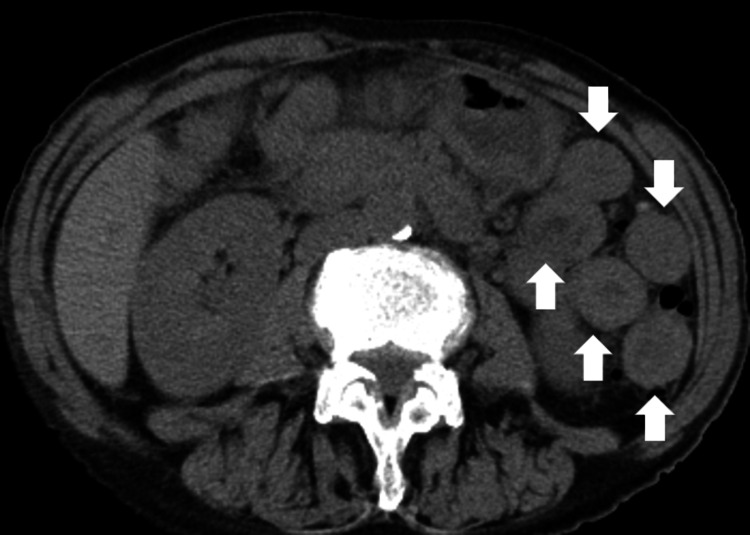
Enterocolitis after avelumab administration in the patient in Case 1 Computed tomography shows diffuse thickening of the small and large intestines (arrows).
